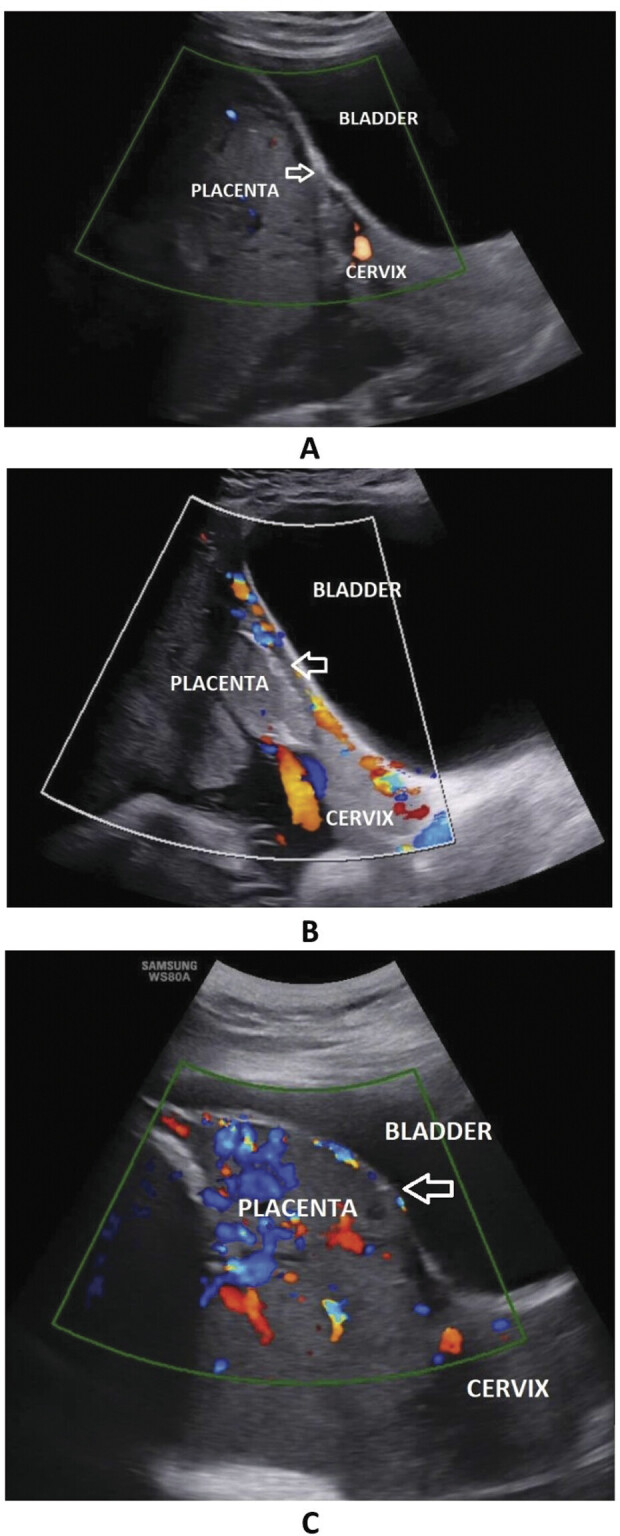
Ultrasound images show the myometrial thickness, vesicouterine interface (white arrow), railway sign, lacunae, placental budge, and the degree of hypervascularity on color Doppler ultrasound. According to imaging characteristics, the types of placenta accreta spectrum disorders are classified as accreta (
